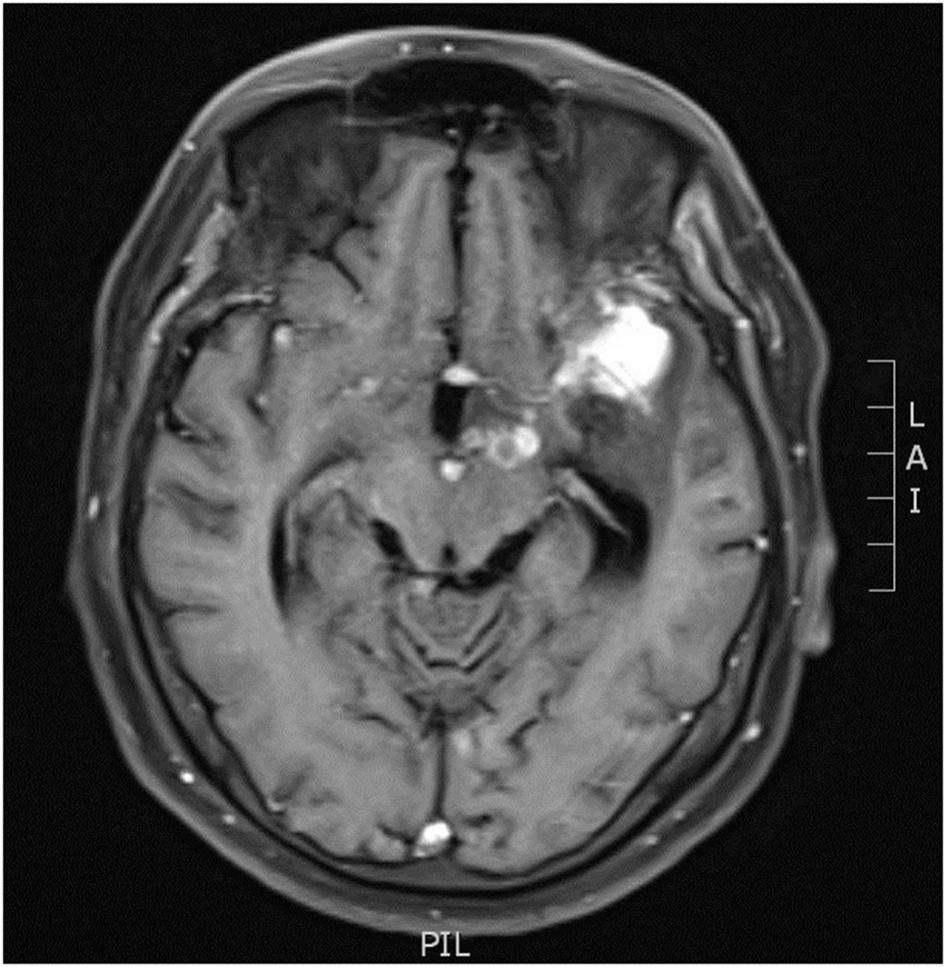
MRI revealed nodular enhancement in the left pontine cistern.
